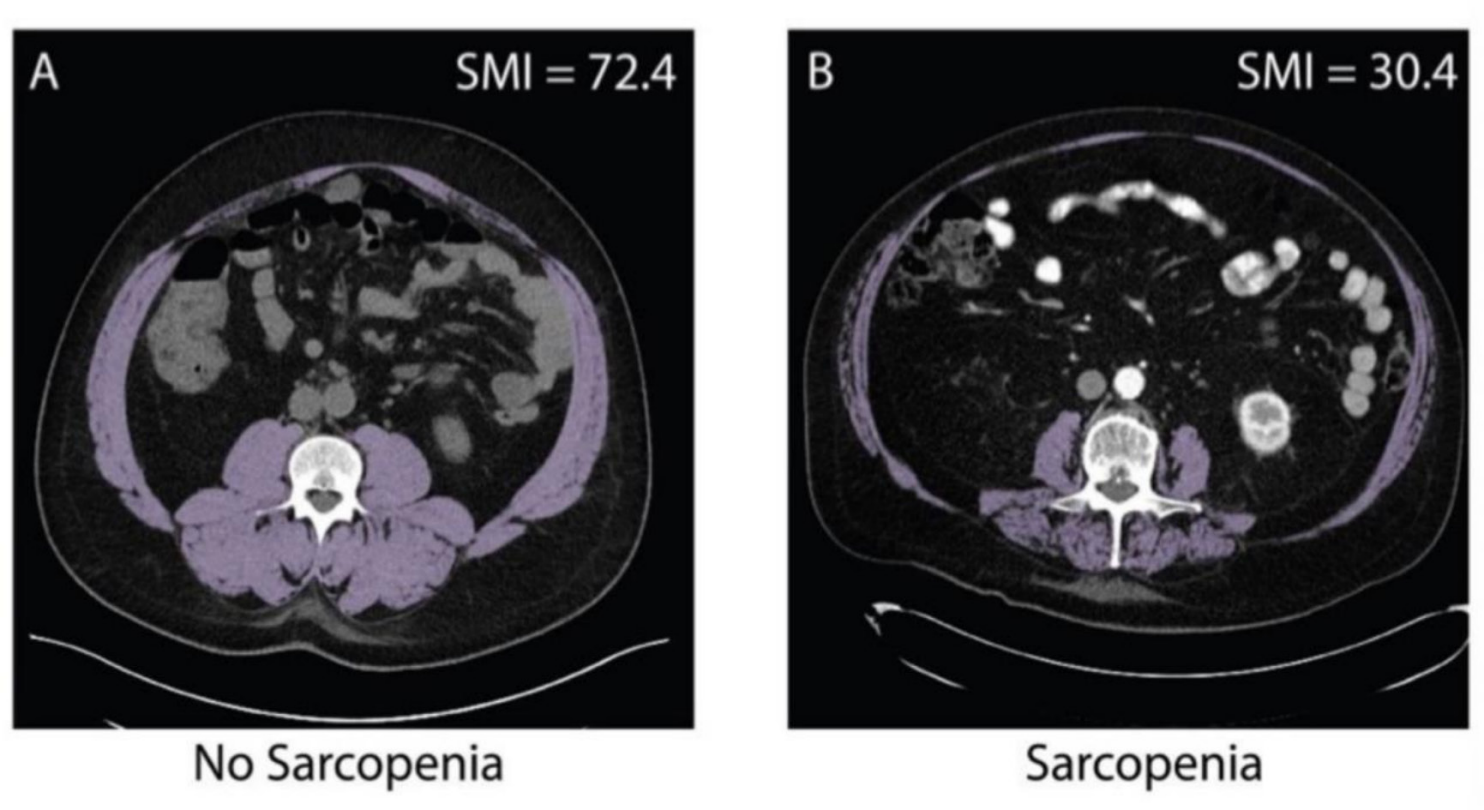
Representative examples of cross‐sectional computed tomography images at L3 level used for the assessment of skeletal muscle mass in (A) a non‐sarcopenic MM patient and (B) a sarcopenic MM patient.
